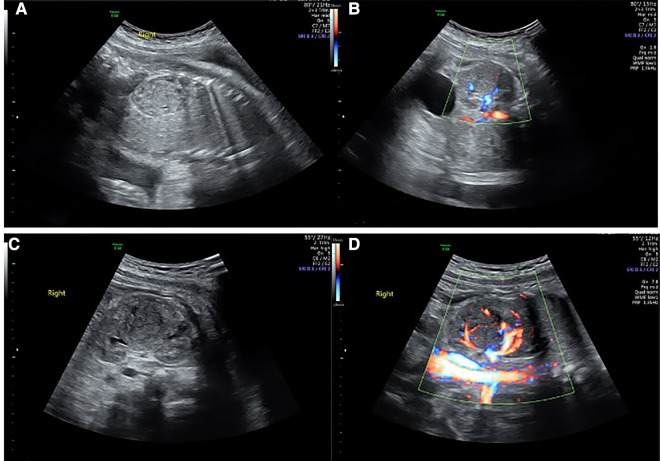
Renal solid mass at different gestational weeks. (A,B) showed the mass at 33 weeks and gestation by 2D and Colour Doppler. (C,D) showed the mass at 36 weeks of gestation by 2D and Colour Doppler.
